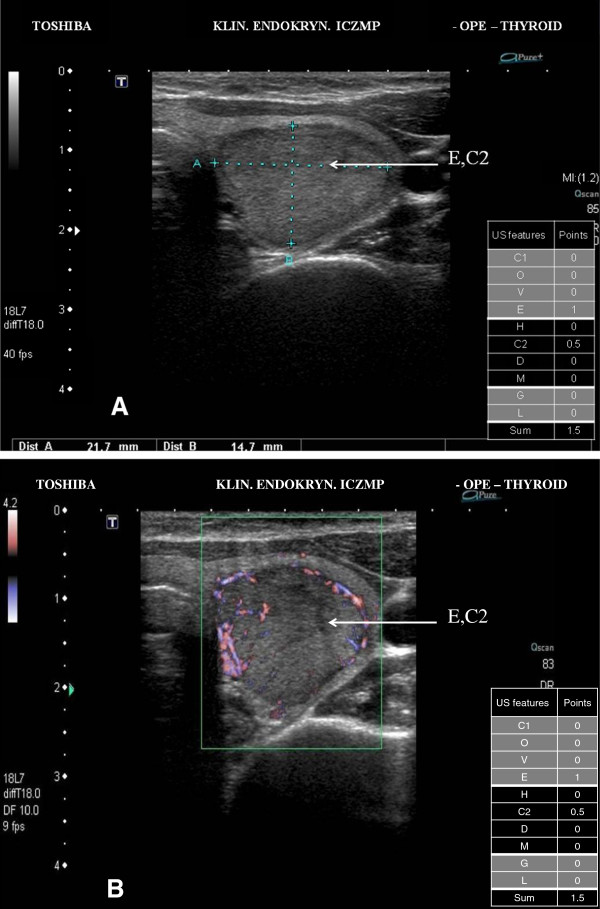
Low risk thyroid nodule/focal lesion US pattern. A. Ultrasound picture of the thyroid lesion (B-mode). The scoring system – 1.5 points (low risk US pattern 0 < 4 points); E – hypoechogenicity; C2 – solid composition; FNAB cytology in this case - category II TBSRTC; management - observation and repeat FNAB examination after 18 months. B. Ultrasound picture of the thyroid lesion (Power Doppler), peripheral blood flows.
